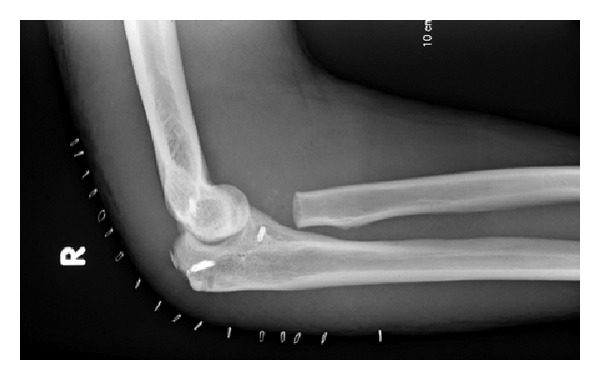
Postoperative X-ray: lateral view showing medial collateral ligament and triceps tendon reconstruction with radial head excision.
